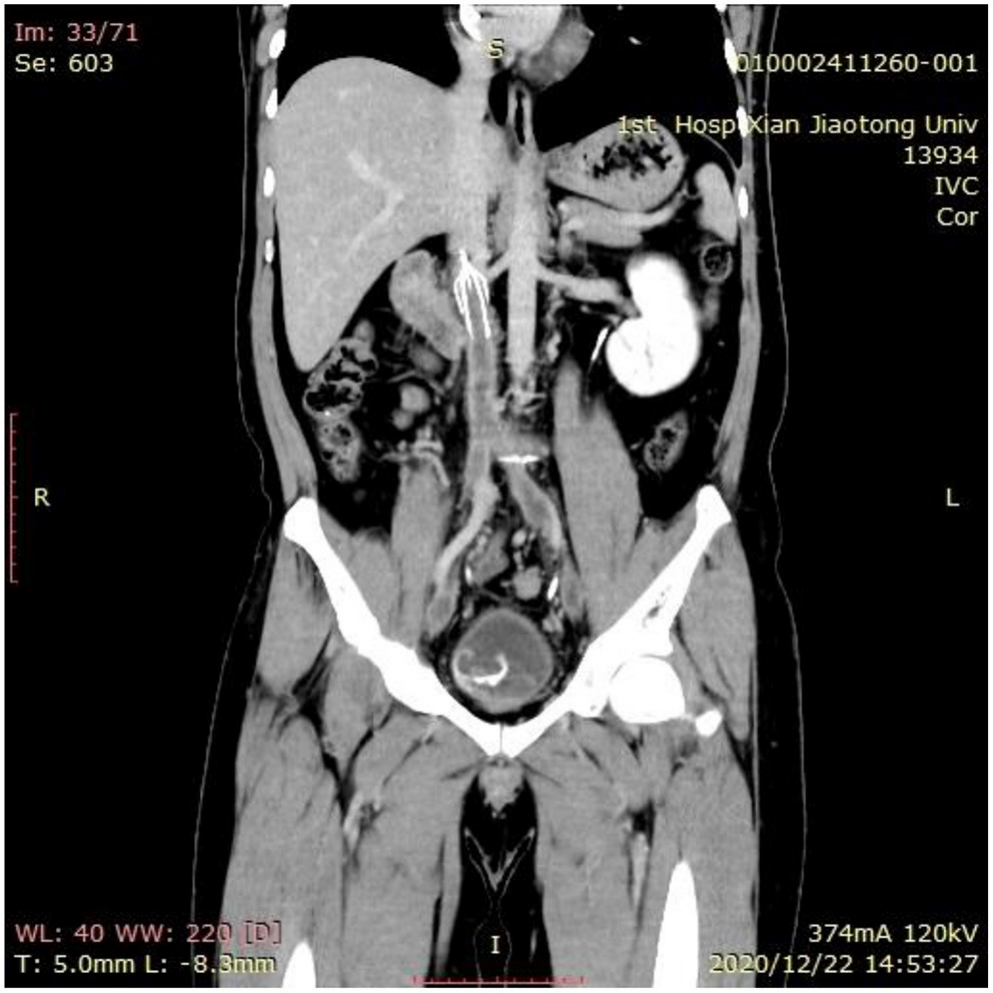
The CT examination of the lower extremity veins showed implantation of the inferior vena cava filter, inferior vena cava and bilateral external iliac vein thrombosis, and bilateral internal iliac vein thrombosis.
